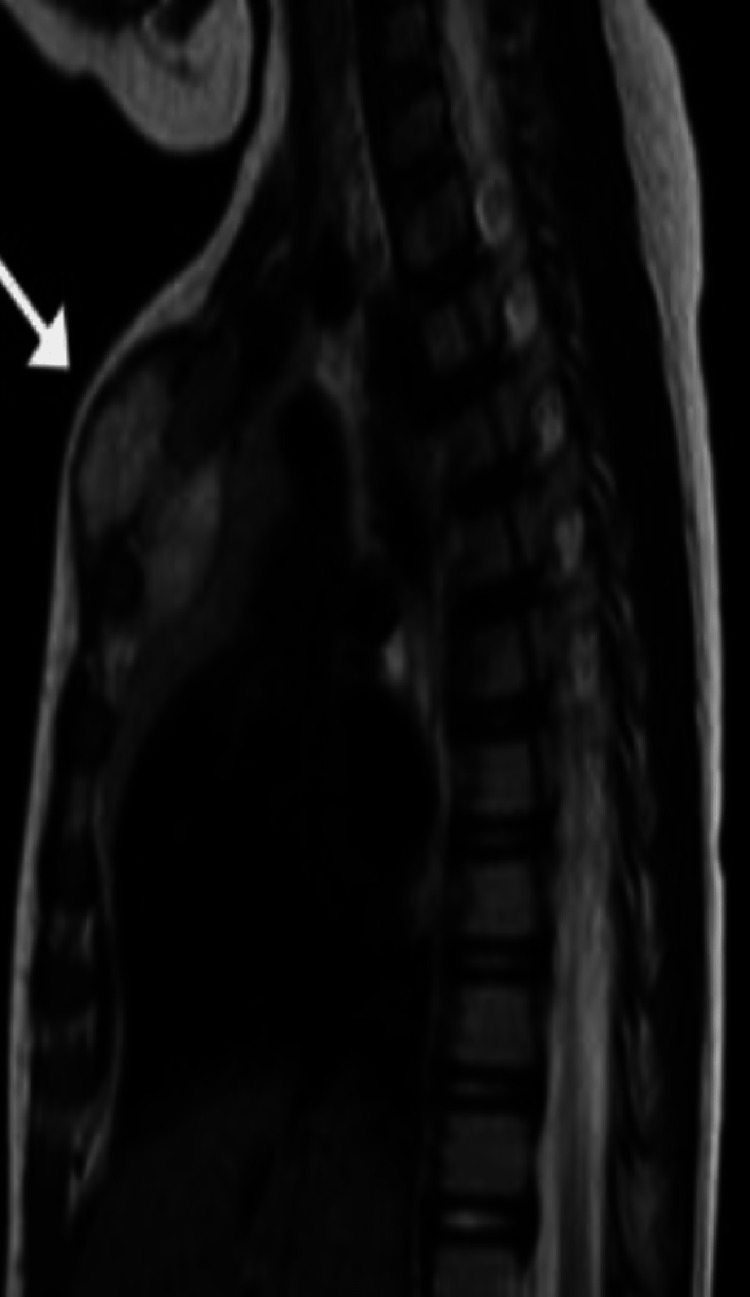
A 15-month-old girl with BCG osteomyelitis The sagittal T2-weighted image shows an encapsulated irregularly shaped abscess, spreading from inside the sternum to both ventral and dorsal surrounding soft tissue [17].
